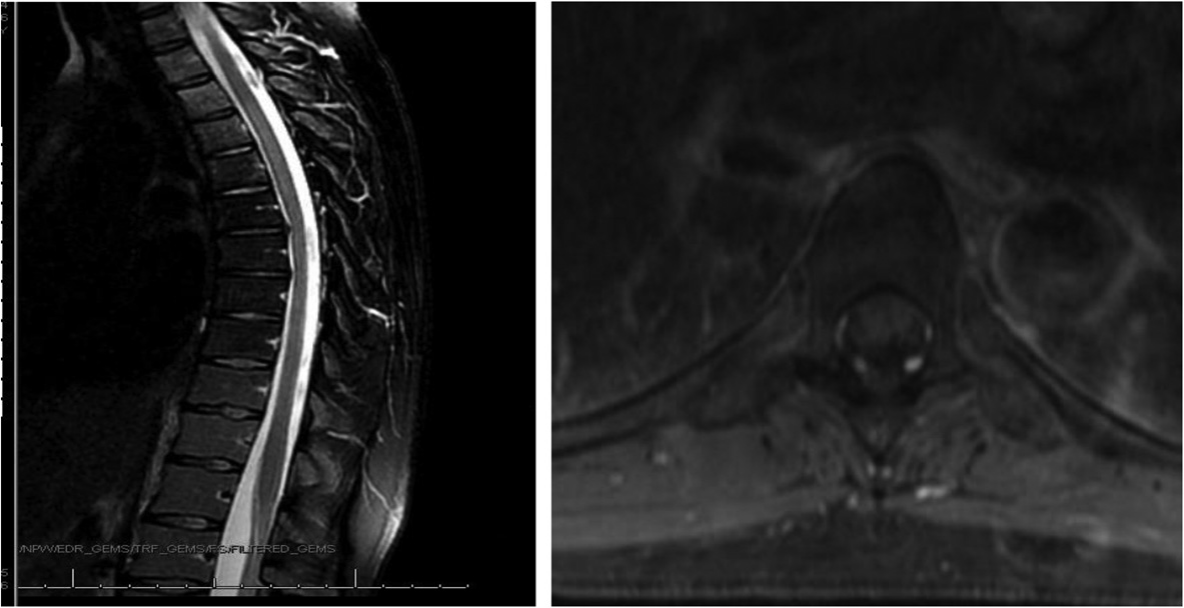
T1-weighted sagittal (left) and axial (right) magnetic resonance images with contrast show dilated subdural veins in a patient with Lhermitte sign and severe symptoms upon walking
